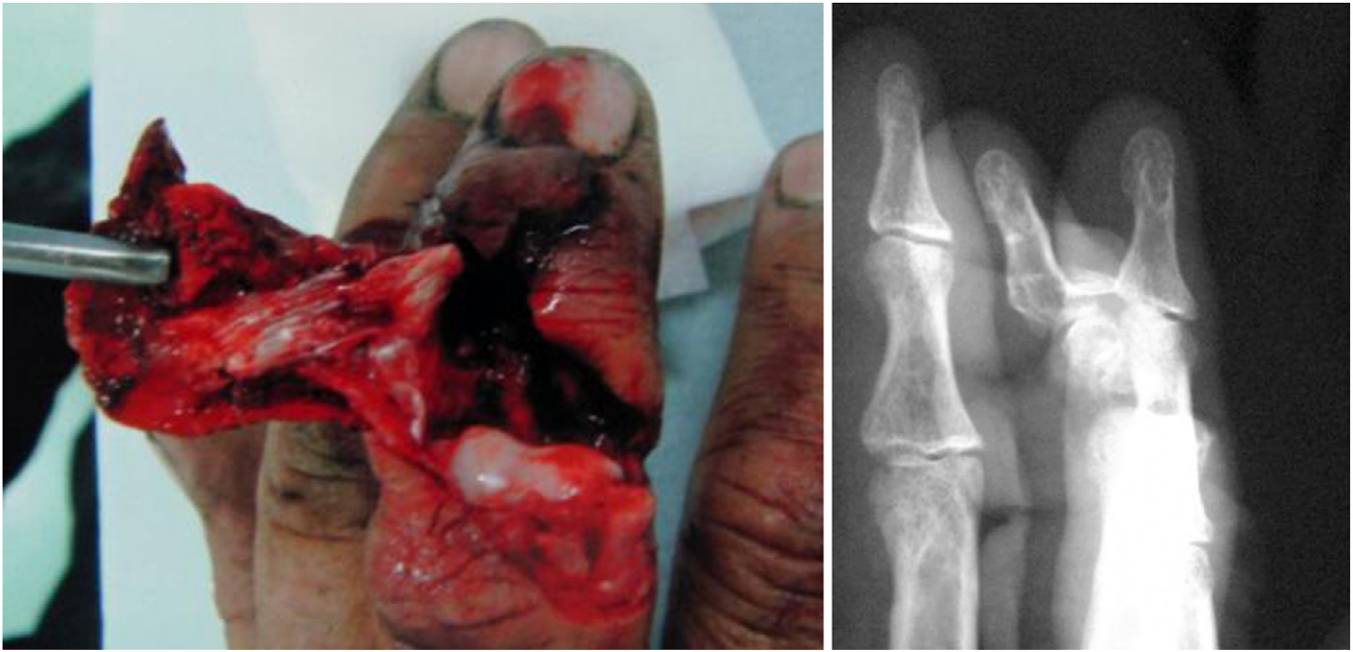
Case 2. (Left) Photograph of the incomplete amputation of the middle finger. (Right) X-ray of the same case. Middle phalange is almost lost.
